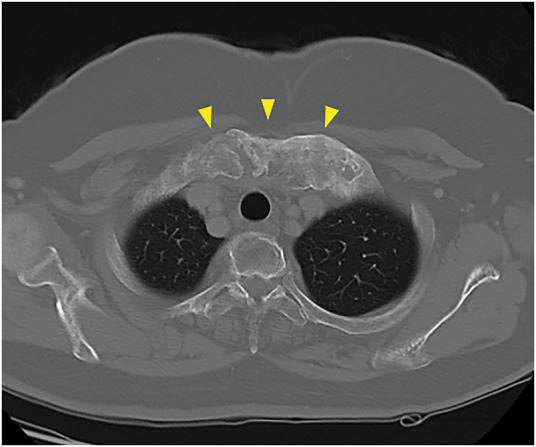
Breast CT image. A tumor with poor contrast enhancement, consistent with a primary lesion, was identified immediately beneath the skin in the C region, with no evidence of metastatic disease. Bilateral sclerosis and hyperplasia of the medial first ribs and sternum were also observed. The wedge markers indicate the lesion.
